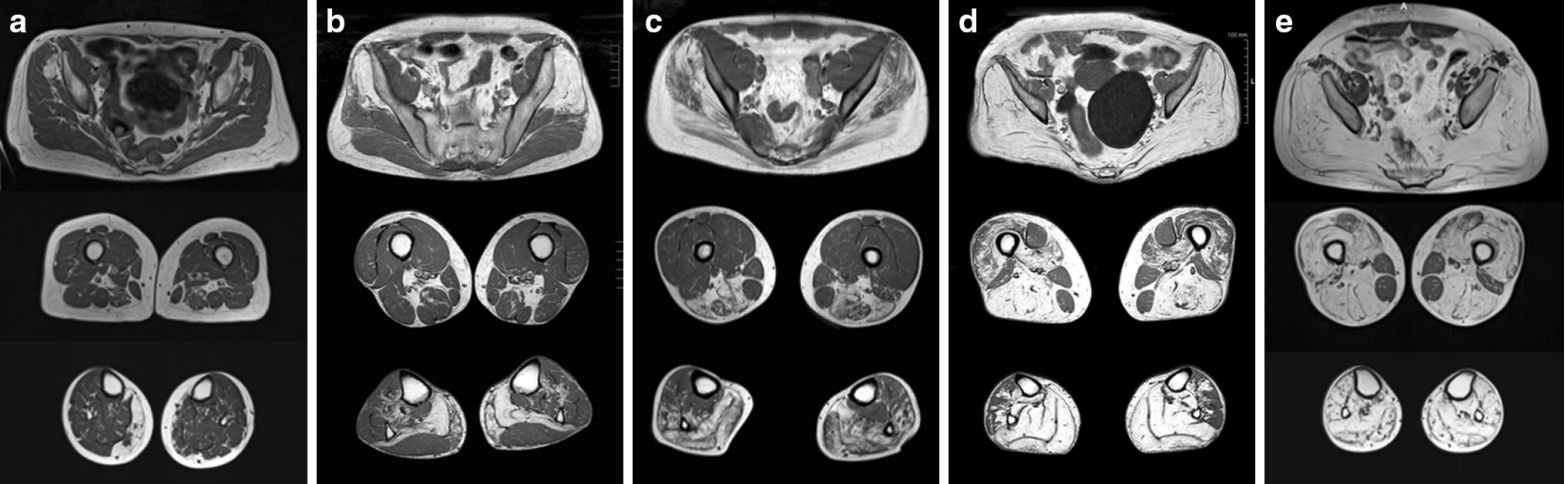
Lower limbs involvement in different stages of NLSD-M. T1-weighted images of lower limbs in NLSD-M patients of different severities: a P10, 74 years; b P1, 62 years; c P9, 25 years; d P6, 52 years; e P4, 45 years. Gluteus minimus, semimembranosus, gastrocnemius medialis and soleus are constantly affected muscles even if in different extents. Leg muscles are invariably more affected than thigh. Posterior compartment of both leg and thigh show more severe involvement than anterior compartment. Psoas, biceps femoris (short head) and rectus femoris show a mild involvement in late-disease course. Gracilis and sartorius are constantly spared
